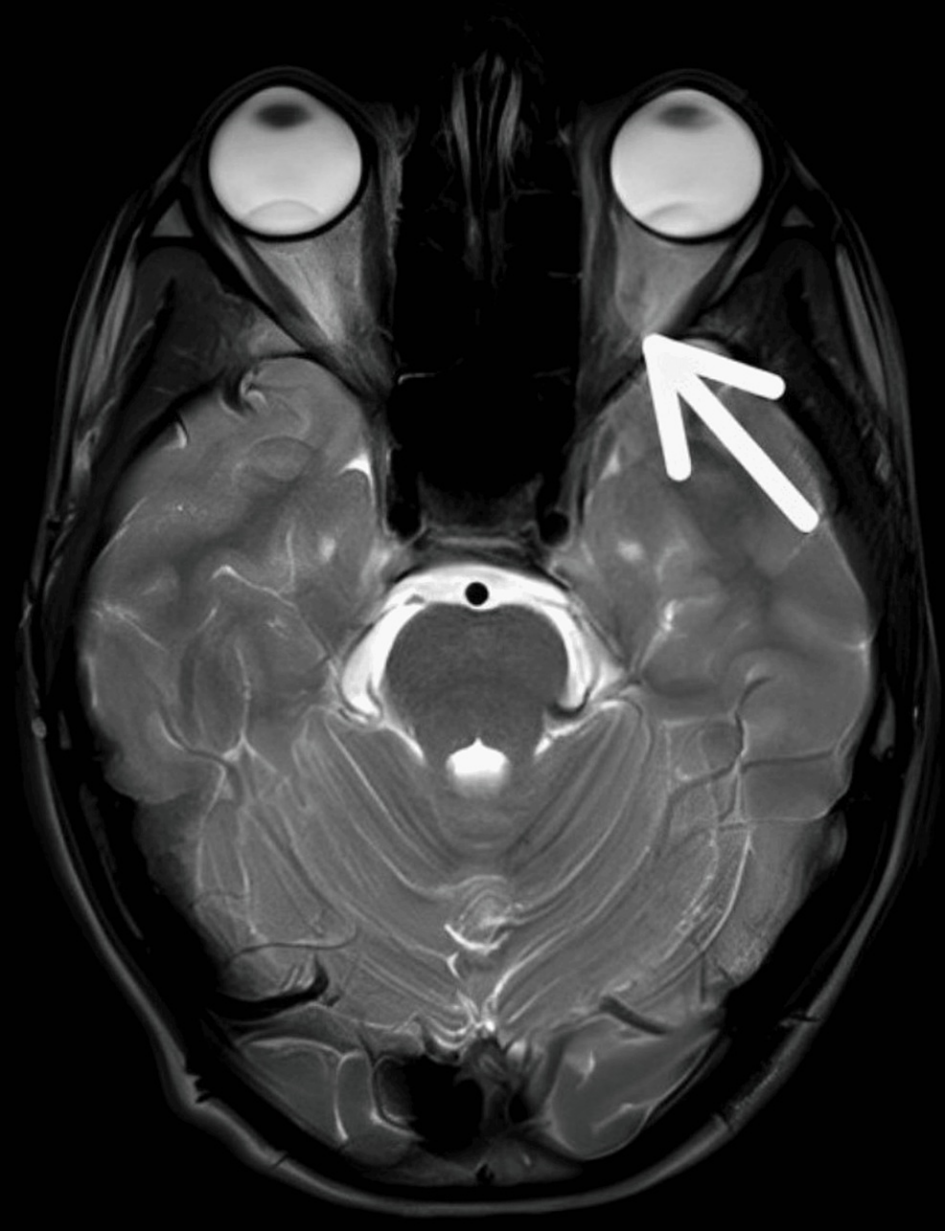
Post-rehabilitation MRI MRI: magnetic resonance imaging
